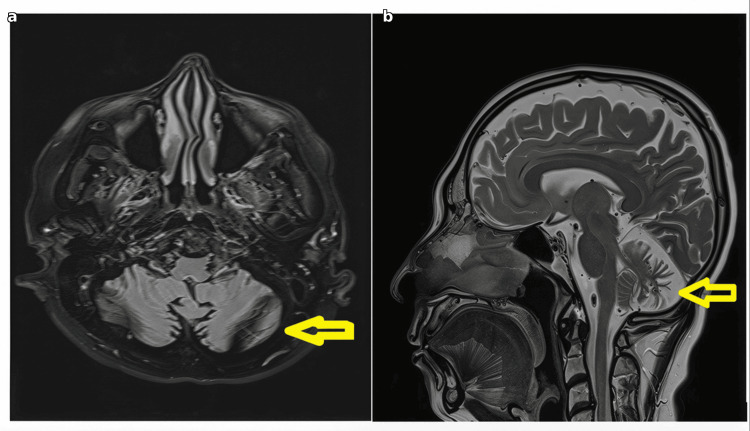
Brain MRI of Case 2 demonstrating cerebellar atrophy (a) Axial T2-weighted image showing cerebellar atrophy with prominence of cerebellar sulci (arrow indicates cerebellar atrophy). (b) Sagittal T1-weighted image demonstrating cerebellar vermian atrophy with enlargement of the fourth ventricle (arrow).
